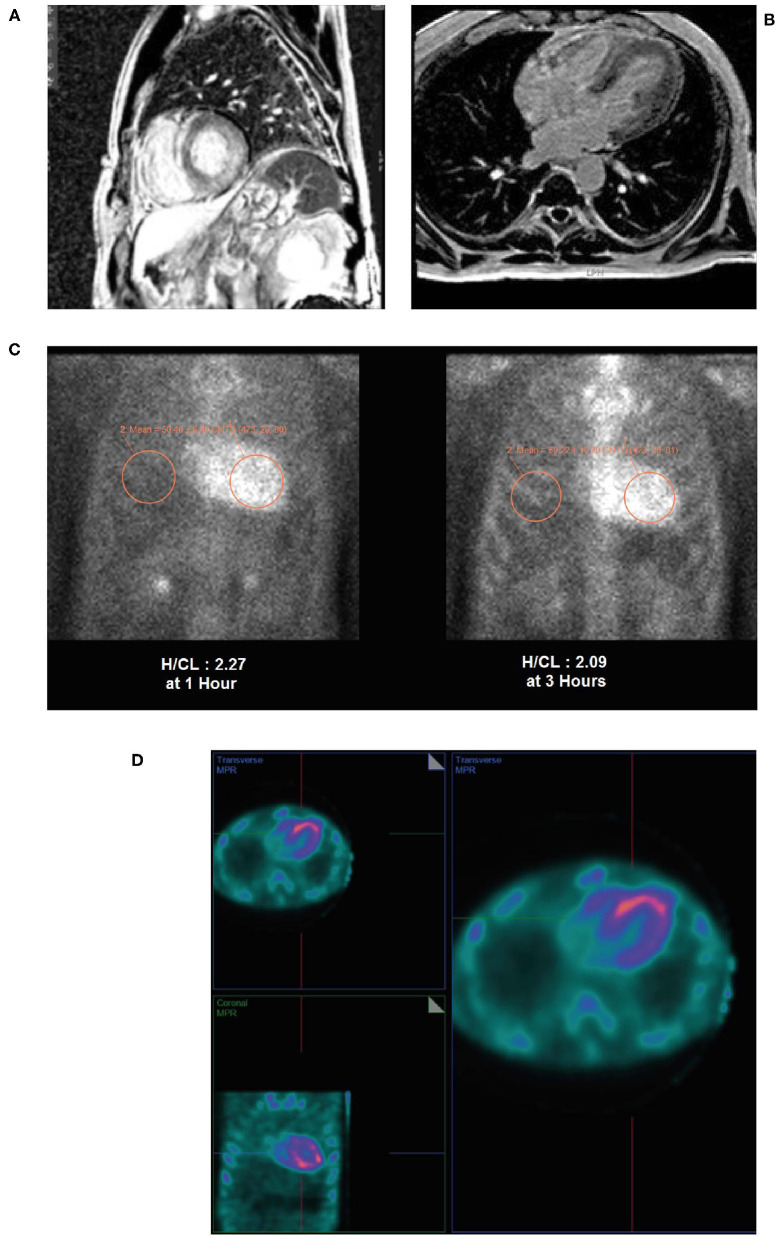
Cardiac MRI (A,B) showing patchy contrast enhancement pattern of the myocardium involving the left ventricular lateral wall base, and mid myocardium of the base to mid ventricle of the LV septum, and mid myocardium of the RV free wall from base to mid ventricle associated with hypertrophy. A planar PYP scan (C) revealed intense cardiac uptake at 1 h and 3 h (H/CL ratio 2.27 and 2.09, respectively), with SPECT scan (D) at 1 h demonstrating tracer uptake in myocardium and no residual LV blood pool activity. H/CL, heart to contralateral lung ratio; LV, left ventricular; MRI, magnetic resonance imaging; PYP, pyrophosphate; RV, right ventricular; SPECT, single-photon emission computed tomography.
